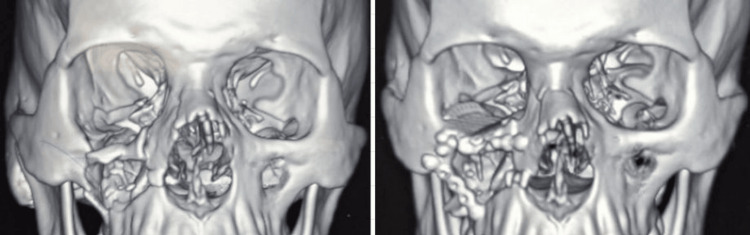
Pre- and post-operative CT image of reconstructed orbit fracture
